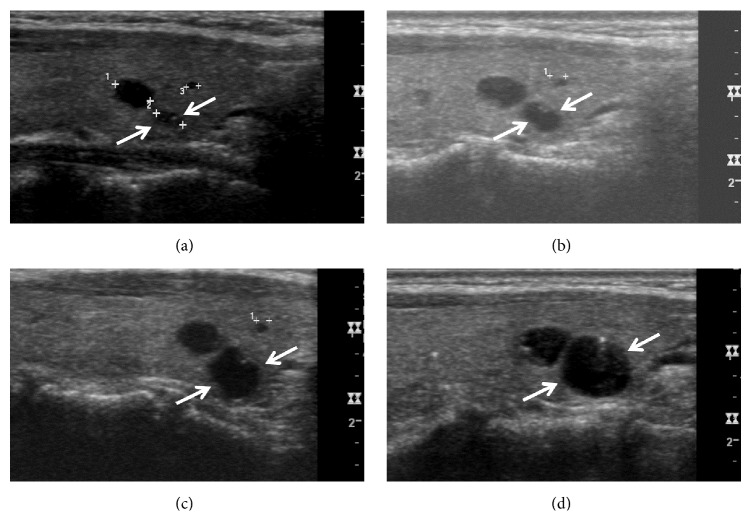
A thyroid colloid cyst with gradual increase in a 50-year-old woman with gradual increase. Longitudinal sonogram (a) shows a colloid cyst with an anechoic nodule and a comet-tail artifact in the right thyroid lobe (arrows, 3.3 mm at its largest diameter). Upon sequential US follow-ups, this colloid cyst demonstrates a gradual increase at a 1-year (b) (arrows, 4.5 mm at its largest diameter), a 2-year (c) (arrows, 6.3 mm at its largest diameter), and a 3-year (d) (arrows, 8.2 mm at its largest diameter) US follow-ups.
